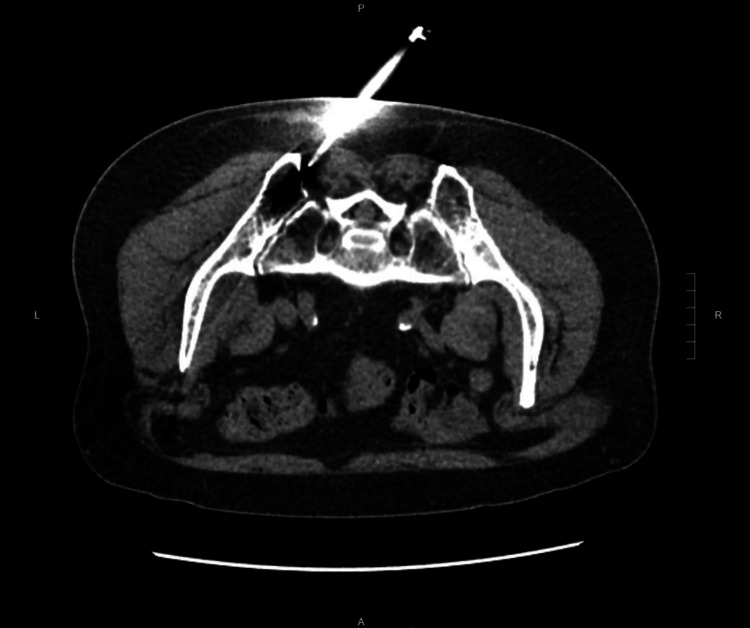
Computed tomography-guided core biopsy of a left iliac crest lesion.
